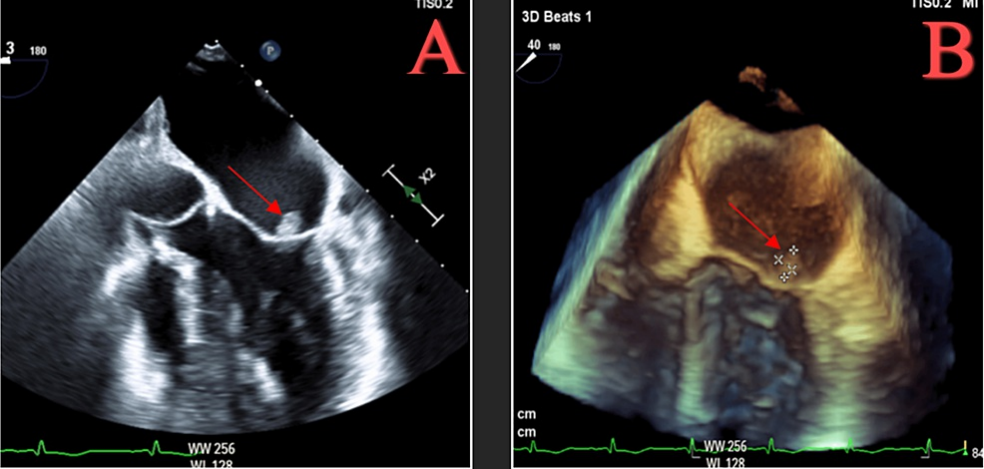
Transesophageal echocardiogram showing 0.7 × 0.4 cm mobile echodensity attached to the posterior leaflet of the mitral valve (A,B).
